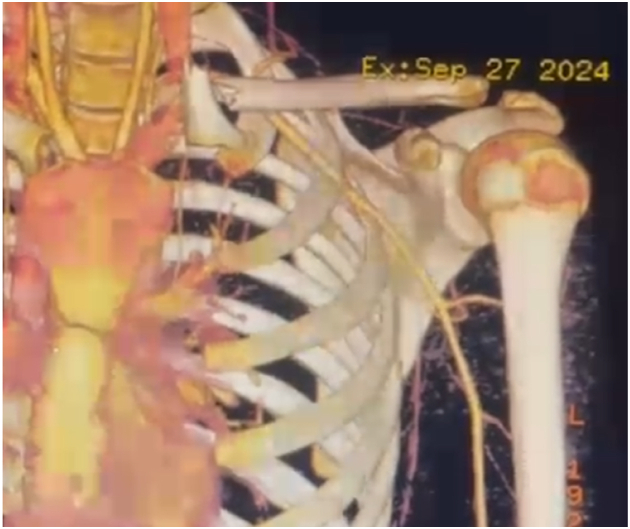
Three-dimensional computed tomography (CT) angiographic reconstruction showing anterior dislocation of the left sternoclavicular joint and 90° rotation of the medial clavicle fragment without evidence of vascular compression.
